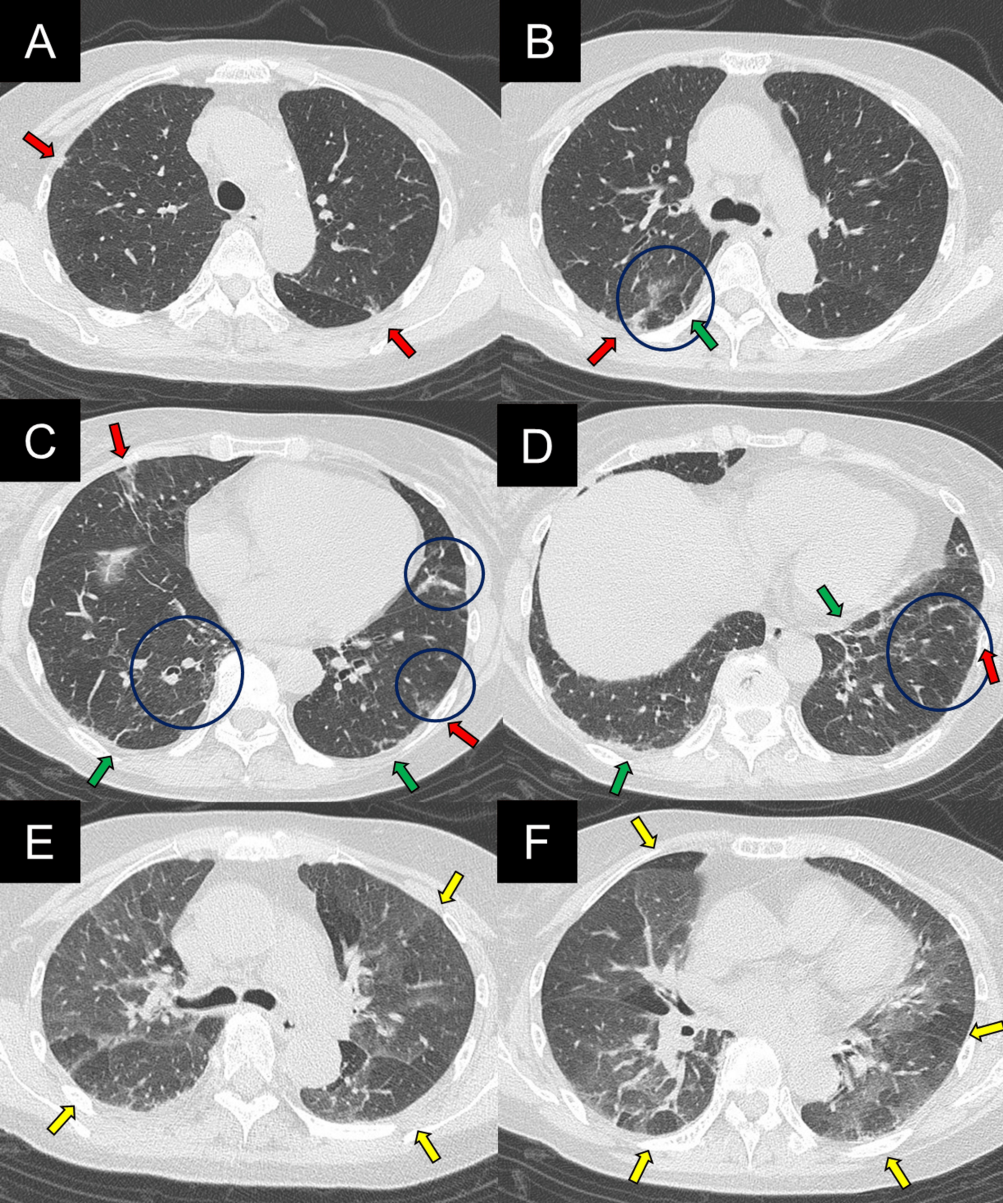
High-resolution computed tomography (HRCT) on admission (A-D) Inspiratory phase images showing diffuse ground-glass opacities (GGOs, red arrows), mosaic attenuation (blue circles), and linear opacities (green arrows). (E-F) Expiratory phase images showing air trapping (yellow arrows).
